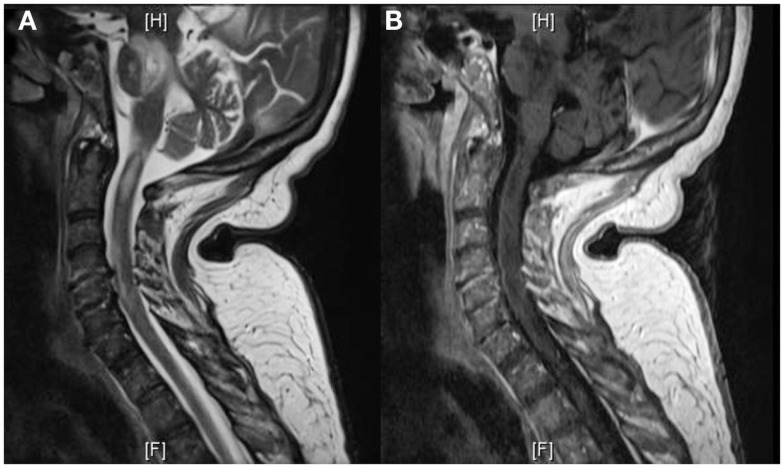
T2W-MRI of the cervical spine shows multiple non-contiguous expansive lesions from the cervico-medullary junction to the level of the sixth cervical vertebra (A). T1W-MRI with contrast demonstrates patchy enhancement (B).
